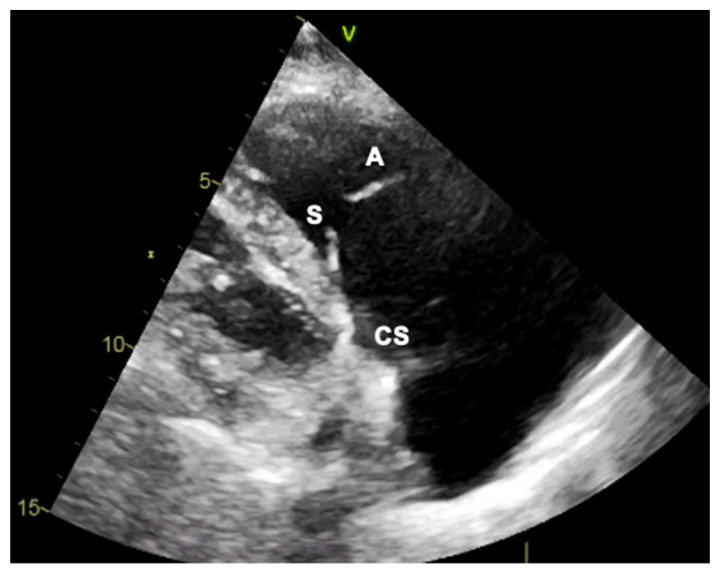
Transthoracic echocardiogram in two dimensions. If the muscular interventricular septum along with the coronary sinus (CS) ostium are seen, then the leaflets visualized are the septal (S) and the anterior (A).
